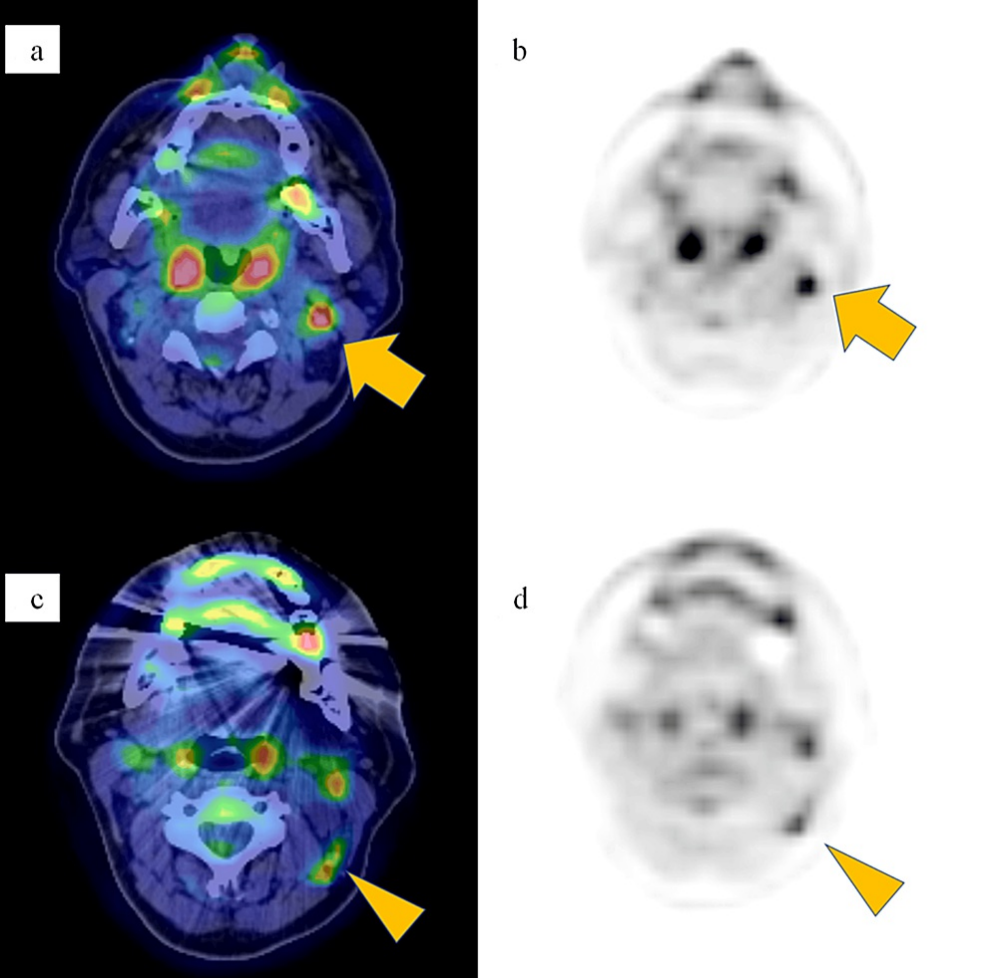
Radiological findings at the initial examination. (a, b) An ^18^F-fluorodeoxyglucose-position emission tomography (FDG-PET) image in the delayed phase/computed tomography, a fusion image acquired in the axial plane, and an FDG-PET image in the delayed phase acquired in the axial plane show ^18^F-fluorodeoxyglucose (FDG) accumulation (standardized uptake value max of 5.3 in the early phase and 6.6 in the delayed phase) at a cervical lymph node (arrow). (c, d) An FDG-PET image in the delayed phase/computed tomography, a fusion image acquired in the axial plane, and an FDG-PET image in the delayed phase acquired in the axial plane show FDG accumulation (standardized uptake value max of 4.1 in the early phase and 4.2 in the delayed phase) at a cervical lymph node (arrowhead).
